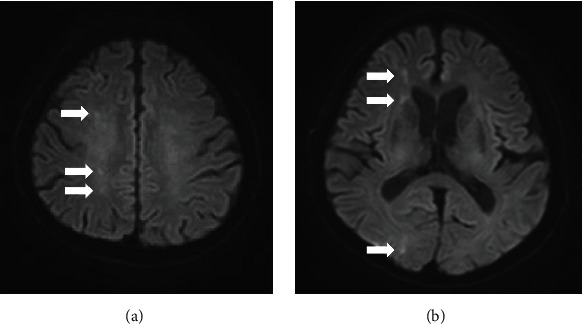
Head diffusion-weighted magnetic resonance images show acute multiple right-hemispheric cerebral infarctions (white arrows).
